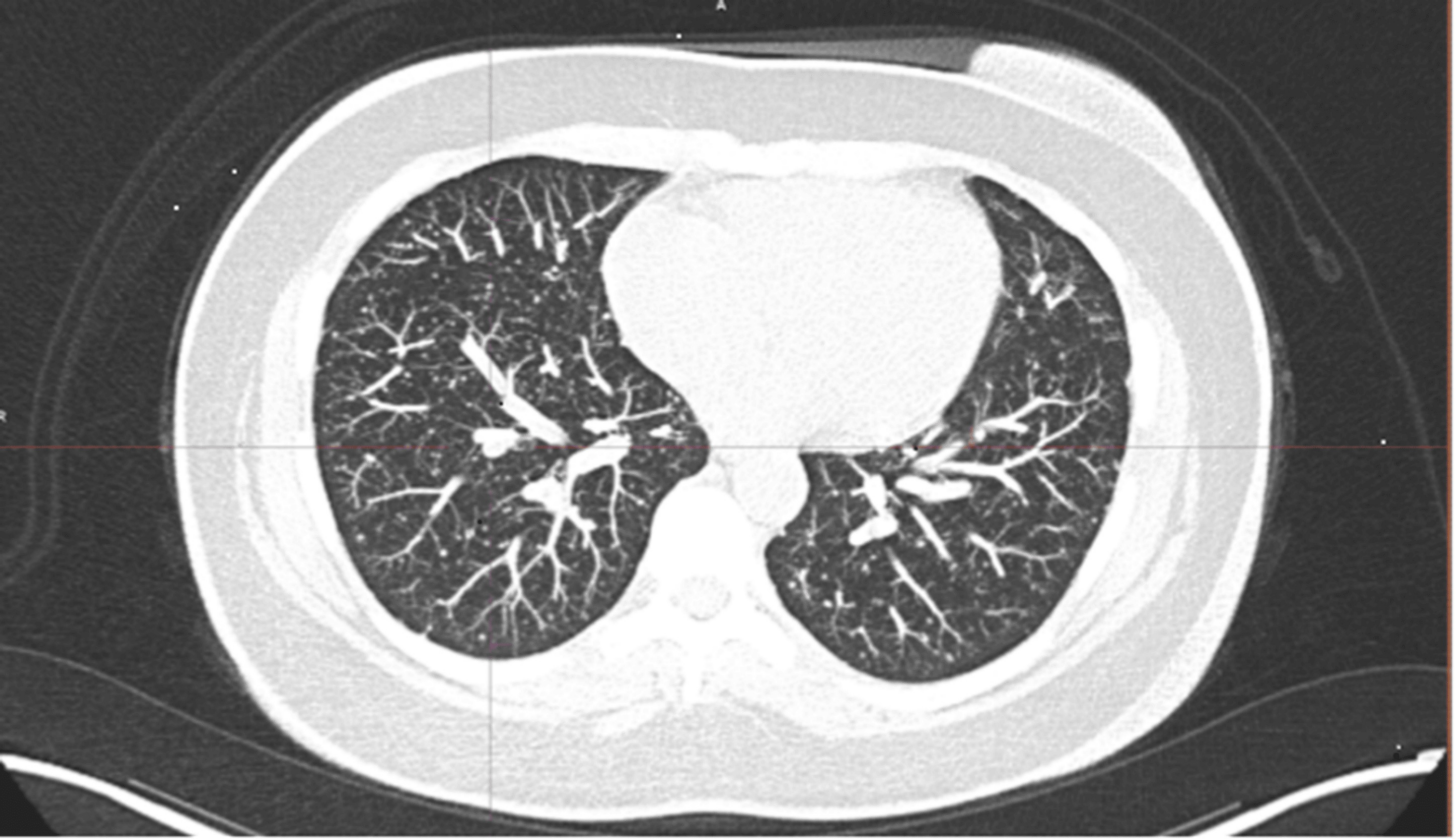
Axial CT slice (lung window), 10 mm MIP reconstruction, showing multiple bilateral pulmonary lesions. CT: computed tomography, MIP: maximum intensity projection.
